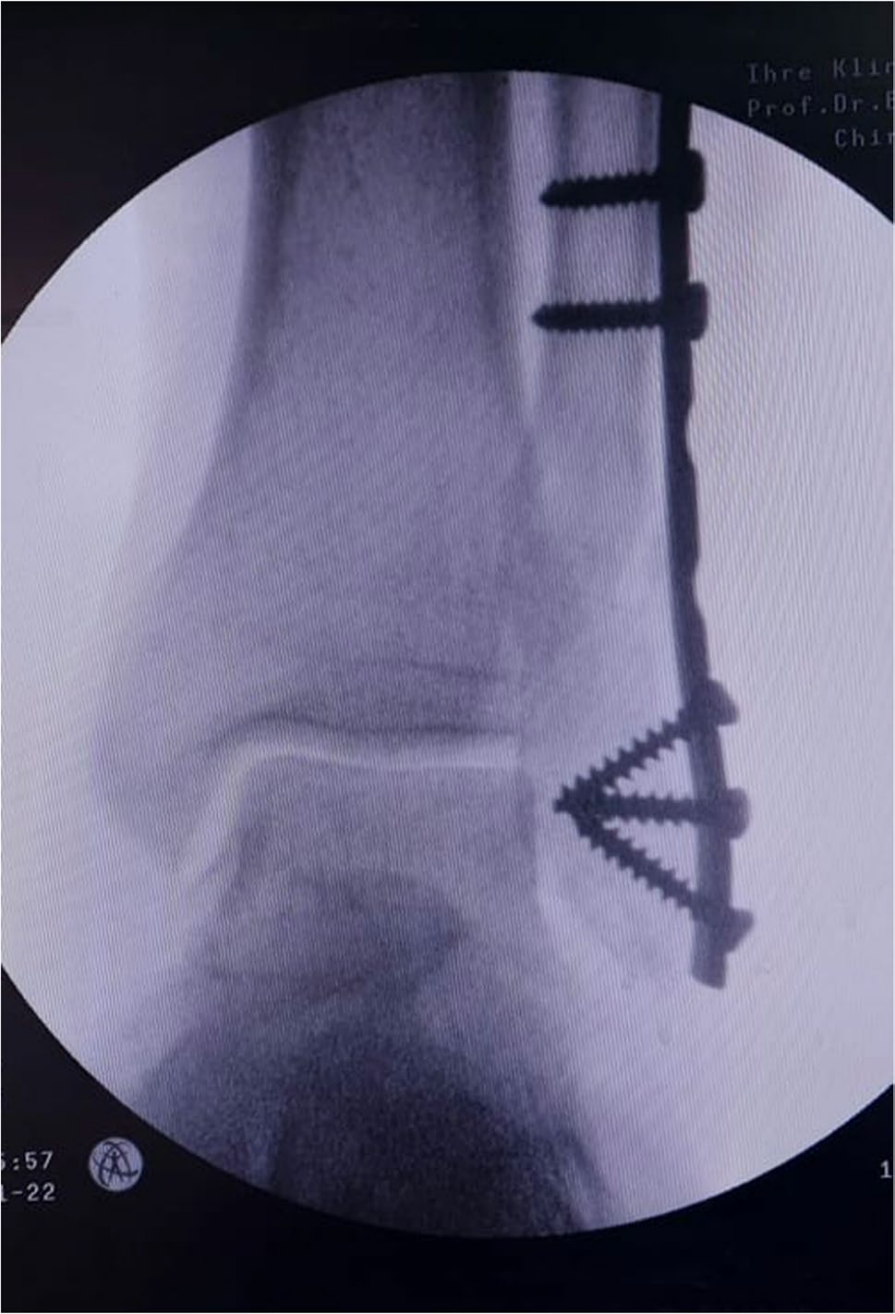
Intra-operative radiographic view after plate fixation
